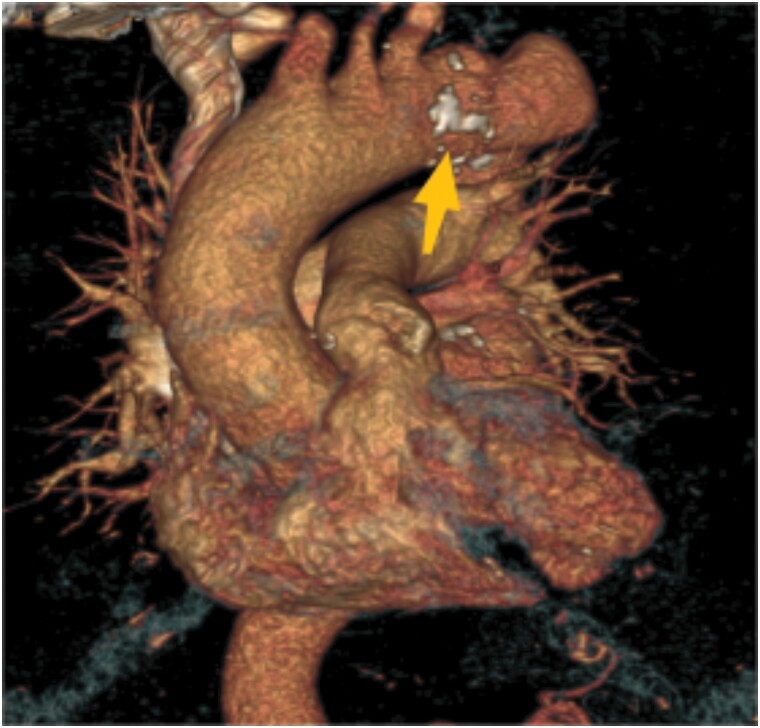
CT angiography with 3D reconstruction of the aorta showing a contained rupture at the proximal anastomosis of the graft.
